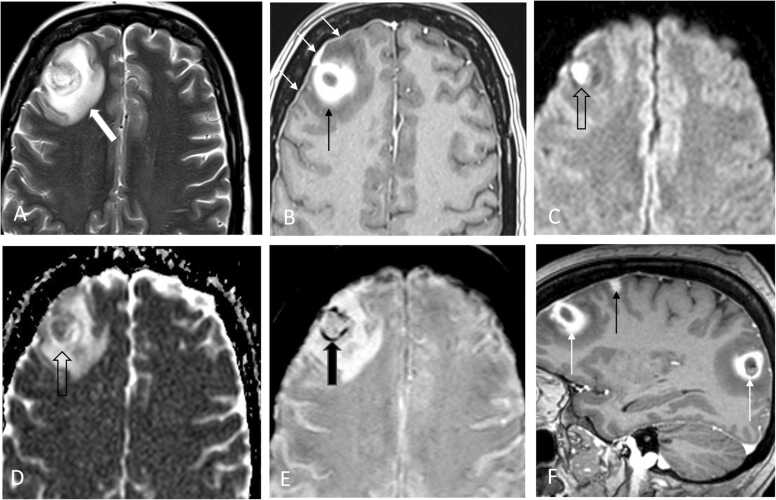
MRI imaging of brain on hospital day 34. Axial T2W (A), contrast enhanced T1W (B), B1000 DWI (C), ADC diffusion map (D), and SWI (E) MRI images of the brain demonstrate a ring enhancing lesion right frontal cortex (black arrow) with associated dural thickening and enhancement over the right frontal cortex (white arrows) and surrounding vasogenic edema (white block arrow). The lesion demonstrated restricted diffusion seen as increased signal on DWI and decreased signal on ADC map images (open block arrow) as well as evidence of petechial hemorrhage seen on SWI (black block arrow). Sagittal contrast enhanced T1W (F) MRI image of the brain demonstrates ring enhancing lesions frontal and temporal lobe (white arrows) and smaller solid enhancing lesion frontal cortex (black arrow).
